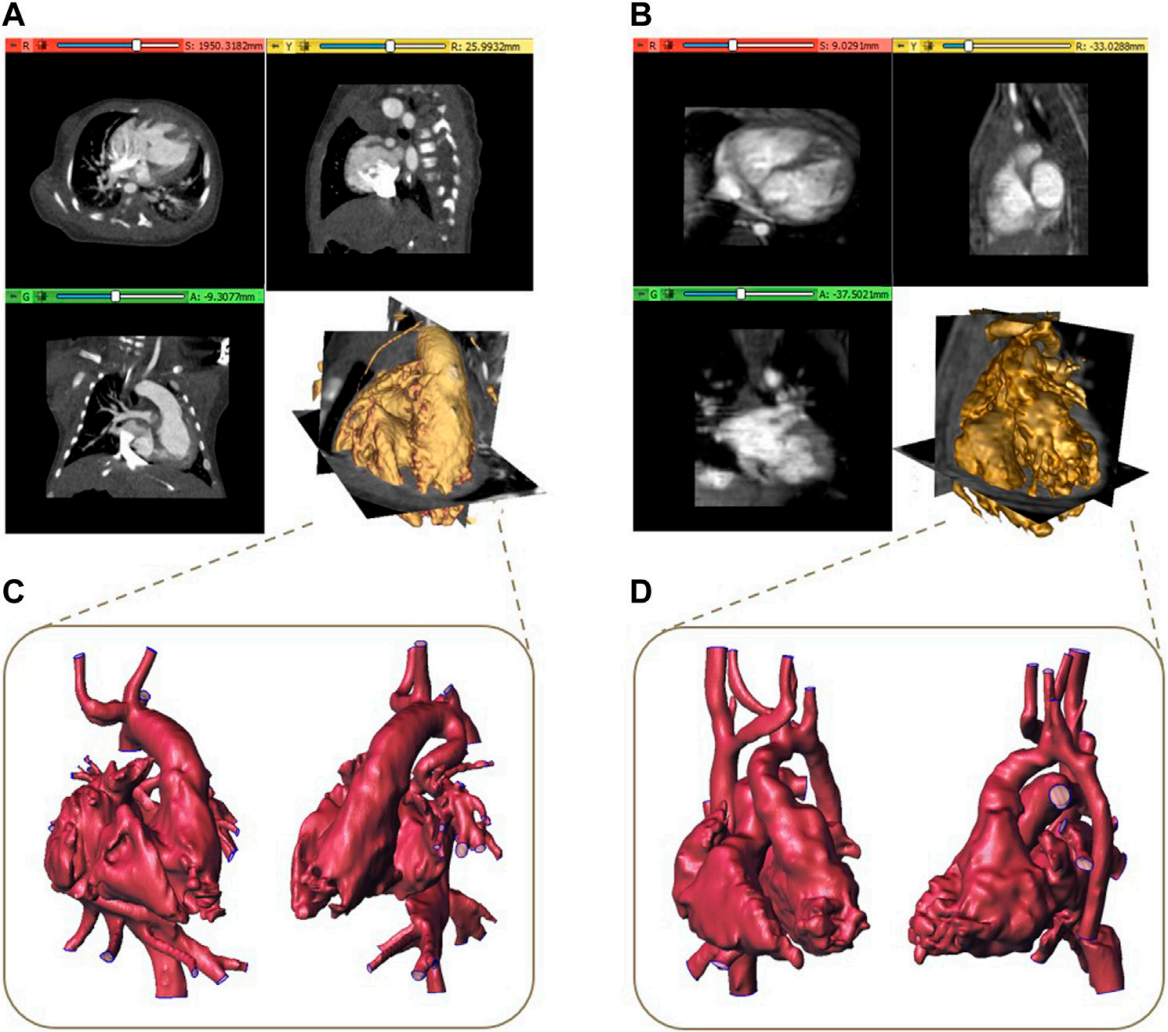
Examples of CT (A) and MR dataset (B) with the associated volume rendering visualization and the corresponding reconstructed 3D models (C, D).
